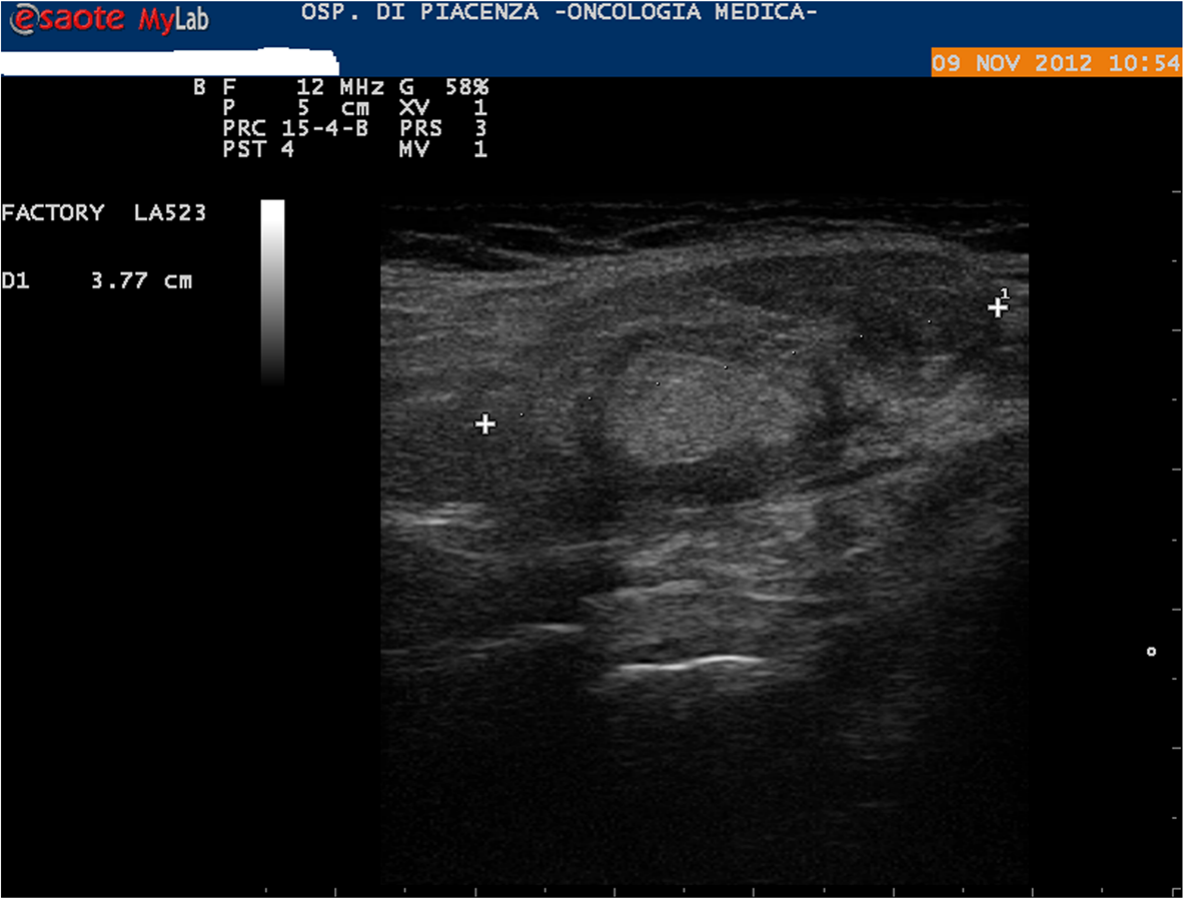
Ultrasound image showing a right submandibular salivary gland enlargement with a low-echogenic pattern.
